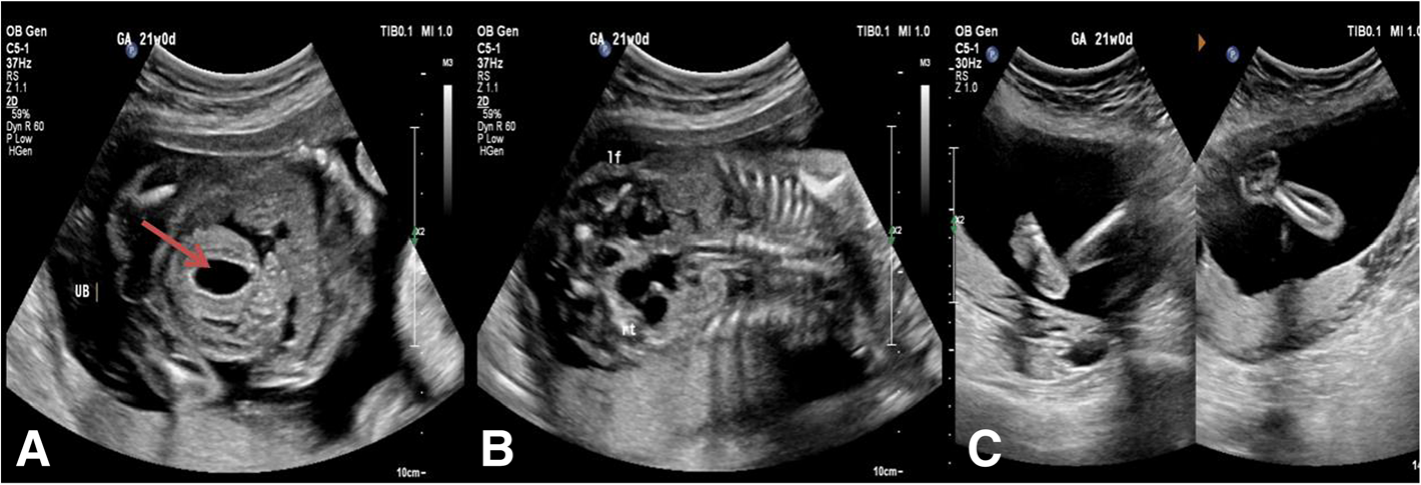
Three images of the first anomaly scan at 21 weeks of gestation in which: a an axial view of two-dimensional ultrasound shows fetal distended urinary bladder wall; b a coronal view of two-dimensional ultrasound shows distended ureters; c an axial view of two-dimensional ultrasound shows bilateral clubfeet. Red arrow is pointing to the fetal urinary bladder
